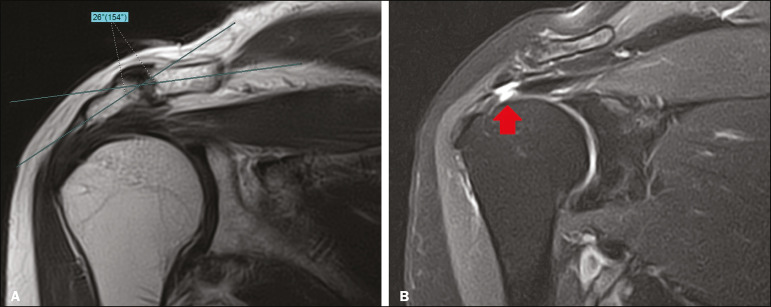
A 77 year-old woman with signs of a supraspinatus tendon transfixing rupture. A: Proton density-weighted image in the oblique coronal plane at the level of the acromioclavicular joint presenting an acromioclavicular angle of 26°. B: T2-weighted image with fat saturation in the oblique coronal plane at the supraspinatus tendon level depicting an interruption of some of the tendon fibers (arrow), characterizing rupture.
